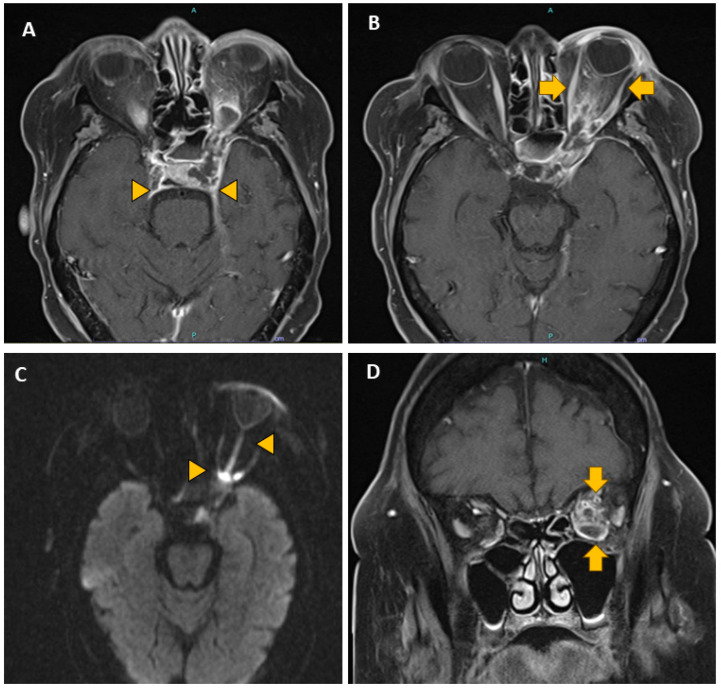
Magnetic resonance imaging, invasive fungal sinusitis with orbital and base of skull involvement. (A). Axial image showing left sphenoid sinus mucosal thickening with internal fluid and erosion of the base of skull (arrowheads). (B). Axial image showing left-sided proptosis and edema in the soft tissues (arrows), extending from the ocular globe to the orbital apex and cavernous sinus. (C). Axial T2-weighted image showing edema of the intraorbital optic nerve (arrow heads). (D). Coronal T2-weighted image showing extensive edema in the posterior orbit (arrows) involving soft tissues, the optic canal, vessels, and oculomotor nerves (orbital apex syndrome).
